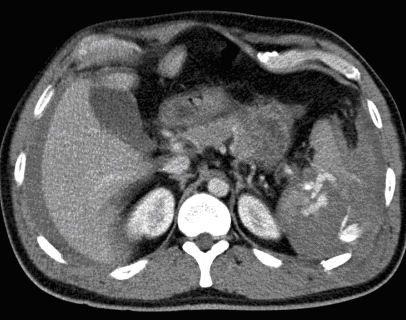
Computed tomography with intravenous contrast showing hemoperitoneum, a fractured spleen with large hematoma and extravasation of contrast medium into the abdominal cavity.
